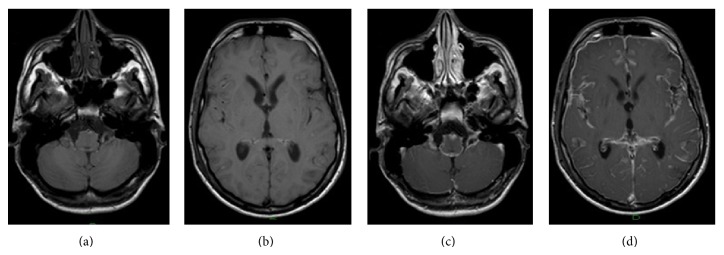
Brain diffuse leptomeningeal melanomatosis in patient 1. (a), (b): T1 weighted axial image (TR/TE 1157 ms/45 ms) shows diffuse sulcal signal hyperintensity due to melanin products which cause T1 shortening signal. (c), (d): contrast-enhanced T1-weighted volume image (TR/TE 25 ms/4,6 ms) shows prominent and extensive leptomeningeal enhancement.
